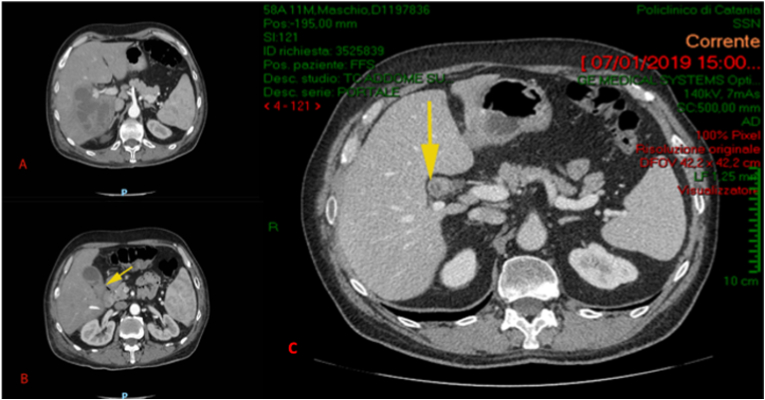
Pre-operative and follow-up CT scan. 1A. Giant hepatic abscess due to intrahepatic gallbladder perforation. 1B. Stone in the cystic stump (yellow arrow) in Mirizzi syndrome. 1C. Follow-up: after four years the stone is in-situ (yellow arrow), retracted in the hepatic hilum, without any sign of inflammation. . (For interpretation of the references to colour in this figure legend, the reader is referred to the Web version of this article.)
